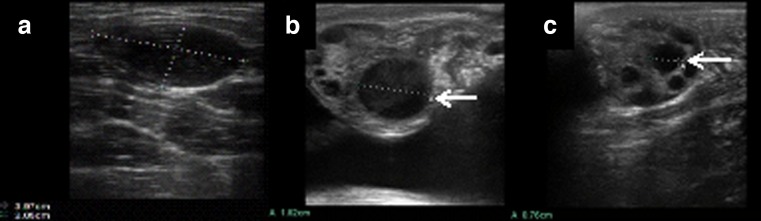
There is no follicle in the ovary via ultrasound detection (a), there is a dominant follicle (18.2 mm, *arrow*) in the ovary via ultrasound detection (b), and there is a small follicle (7.6 mm, *arrow*) in the ovary via ultrasound detection (c)
